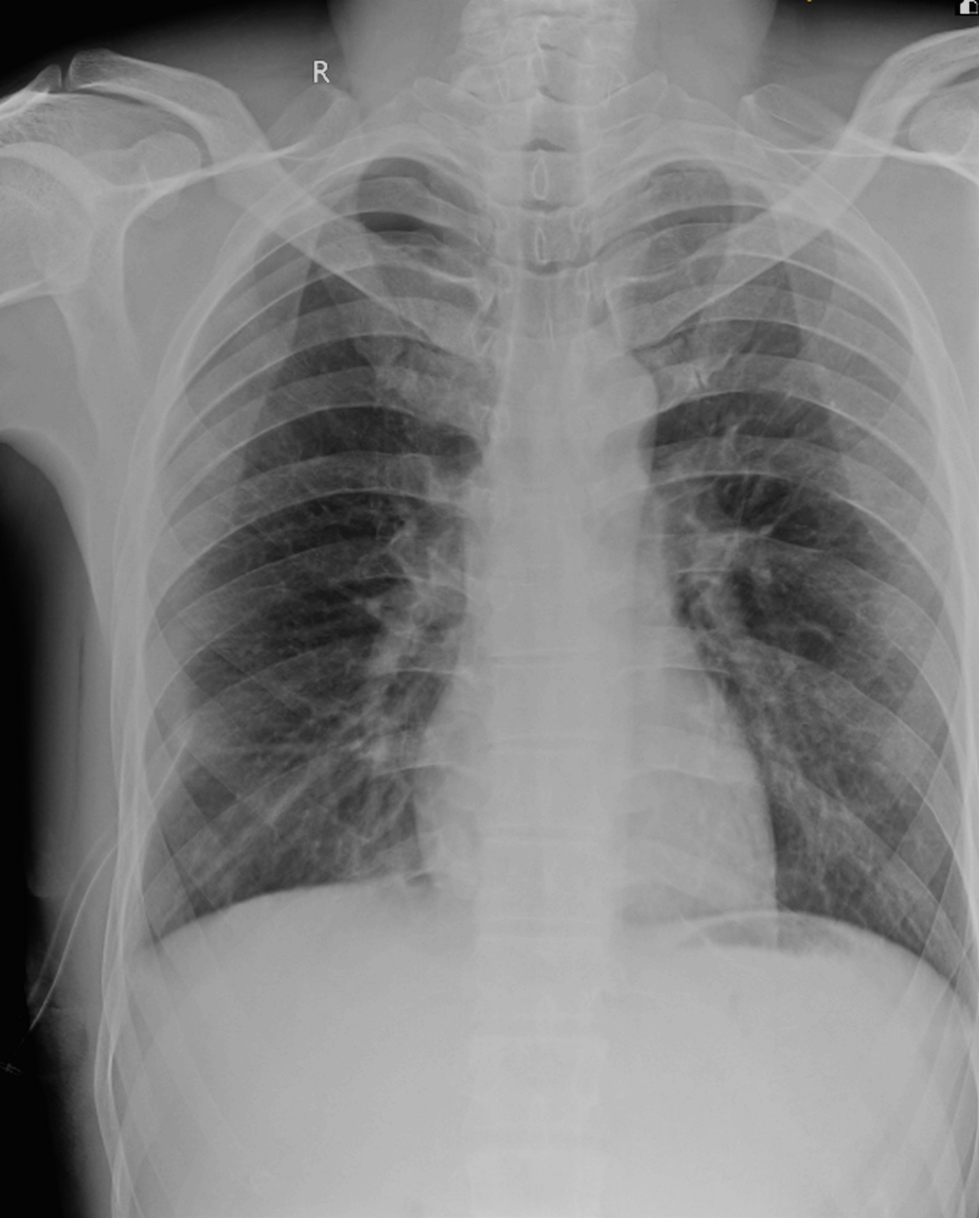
Chest X-ray (PA view) showing complete radiological resolution of pneumothorax with an intercostal drain in situ on the right side. PA, posteroanterior
